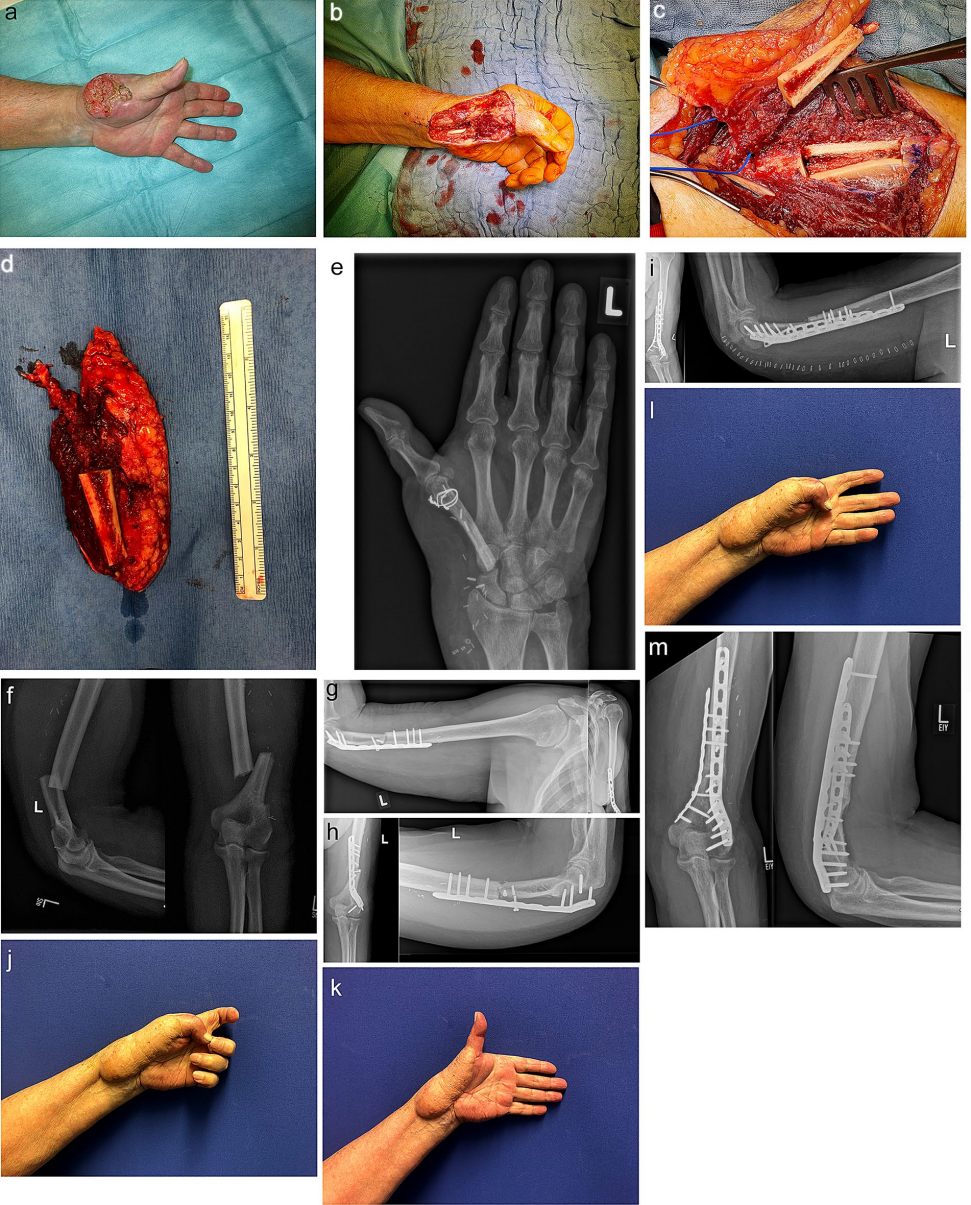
a Before radical resection. b After radical resection. c Free osteocutaneous LAF before elevation. d Free osteocutaneous LAF after elevation. e X-ray 12 months after initial operation. f Humerus fracture. g Humerus fracture after plate osteosynthesis. h Plate and screw dislocation after initial osteosynthesis. i X-Ray after cancellous bone transplantation. j–l Final result achieved 12 months after reconstruction. m X-Ray 7 months after resection of the pseudarthrosis and spongioplasty
